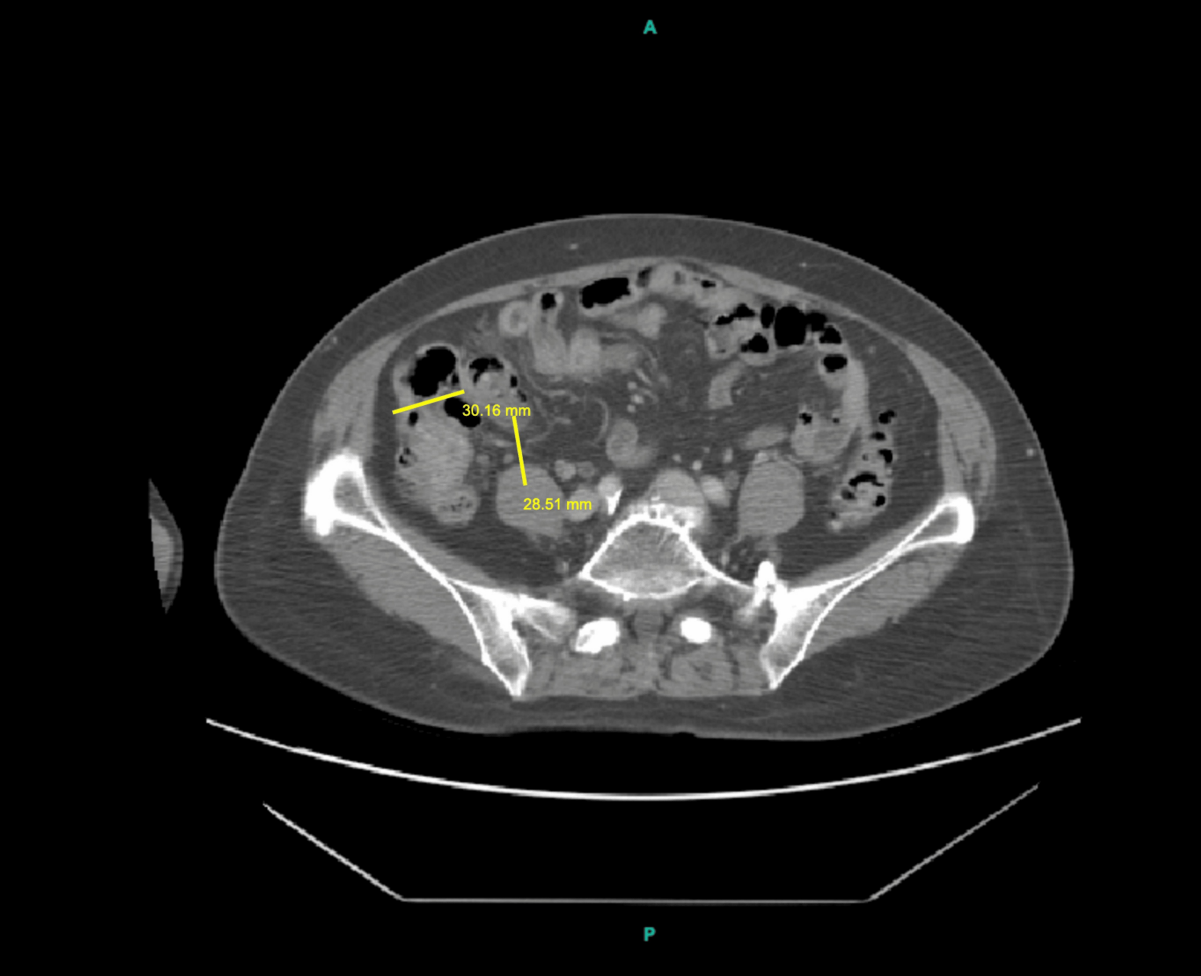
An axial CT scan of the abdomen demonstrating the ascending colon with a suspected polypoidal lesion. The yellow lines correspond to the dimensions of the polyp: the 30.16 mm line (anterior to the polyp) represents the transverse (left-right) axis, while the 28.51 mm line (medial to the polyp) represents the AP axis. CT: computed tomography; AP: anteroposterior
